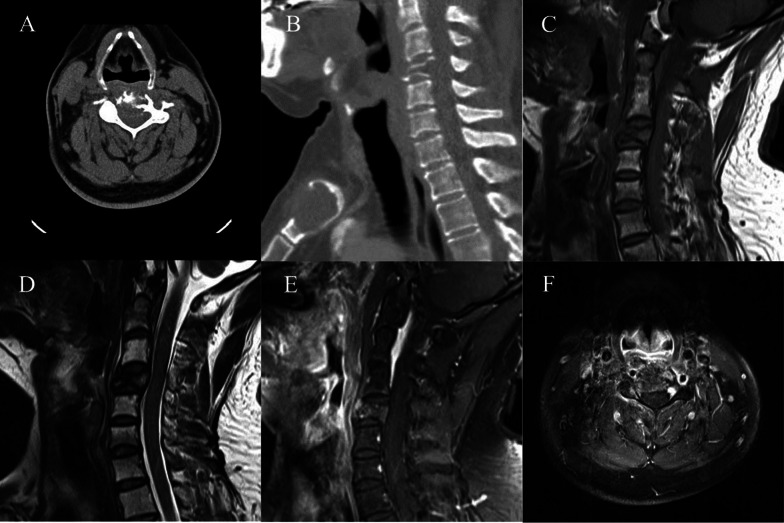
A 37-year-old man with EHE (case 4). a Axial CT showing an ill-defined expansile osteolytic lesion of C4 without a sclerotic margin, containing thickened residual bone trabeculae. b Sagittal CT showing multiple lesions of the manubrium and C4 vertebra. c MR T1WI sagittal view showing the intermediate SI of the lesion. d T2WI sagittal view showing that the tumor has slightly high SI. e Enhanced MR scan in the sagittal view showing heterogeneous enhancement of the mass. f Enhanced MR scan axial view showing that the mass had extended into the posterior elements
